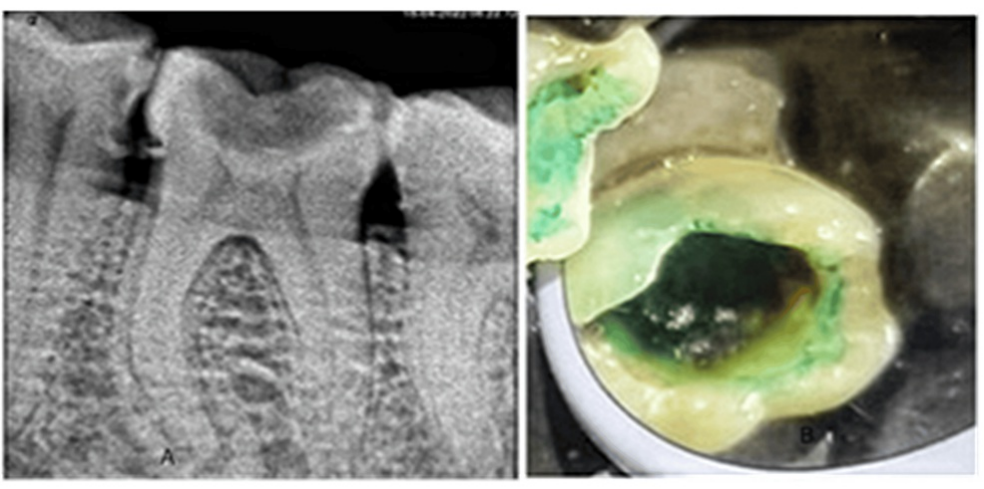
A shows pre-operative IOPA and B shows the application of caries indicator dye IOPA: intraoral periapical radiograph
